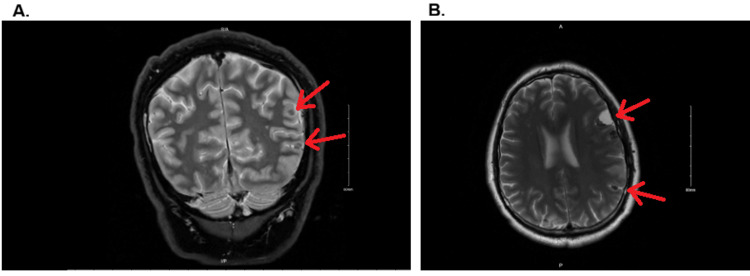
A-B: MRI brain with and without contrast showing peripherally enhancing cystic lesions in the left frontal cortex and right posterior parietal cortex, consistent with the vesicular (early) stage of neurocysticercosis. The lesions exhibit mild peripheral enhancement, small nodular components, and minimal surrounding edema. Smaller calcified granulomas, indicative of the calcified (late) stage, are also seen.
